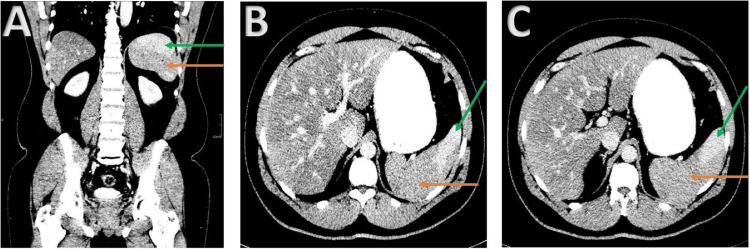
Contrast enhanced computed tomography (CECT) images obtained 12 hours after symptom onset Coronal (A) and axial (B and C) CECT images in the portal venous phase demonstrate abnormal enhancement of the spleen with a large non-enhancing area within the posterior and inferior spleen (orange arrows). Normal enhancing spleen is noted anteriorly (green arrows).
